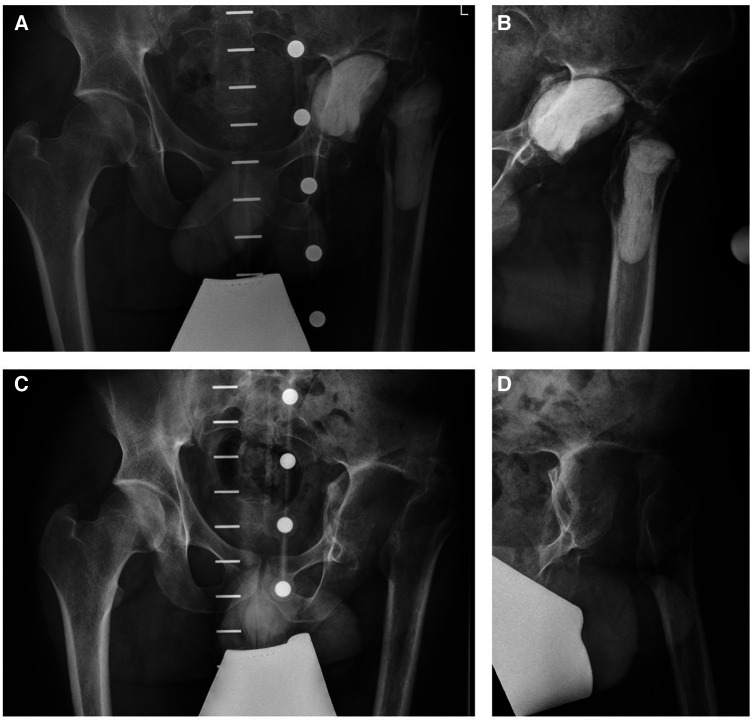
Pelvic x-rays (A,B) showing the customized cemented filler containing gentamicin, vancomycin and cefiderocol. Foreign body removal was performed before hip arthroplasty implantation (C,D).
